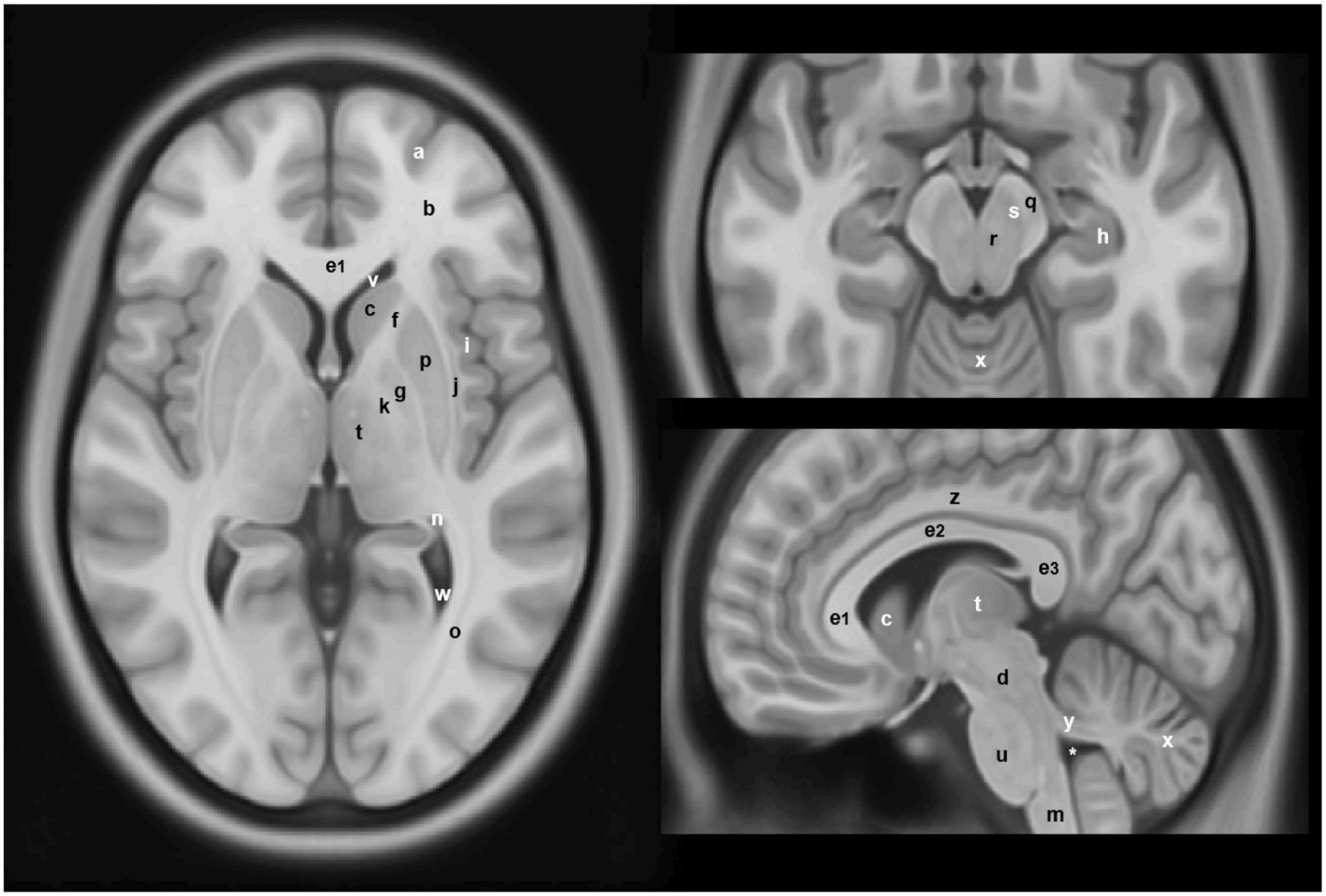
Anatomical locations of some of the structures and regions important in Parkinson's disease and atypical Parkinsonian syndromes, highlighted on a standard averaged T1-weighted MNI template for normal population. Labeling: a = cerebral gray matter (frontal lobe), b = cerebral white matter (frontal lobe), c = head of caudate nucleus, d = midbrain, e1 = genu of corpus callosum, e2 = body of corpus callosum, e3 = splenium of corpus callosum, f = anterior limb of internal capsule, g = globus pallidus, h = hippocampus, i = insular cortex, j = claustrum, k = posterior limb of internal capsule, m = medulla oblongata, n = tail of caudate nucleus, o = optic radiation, p = putamen, q = crus cerebri (anterior portion of cerebral peduncle), r = red nucleus, s = substantia nigra, t = thalamus, u = pons, v = anterior horn of lateral ventricle, w = posterior horn of lateral ventricle, x = cerebellum, y = superior cerebellar peduncle, z = cingulate gyrus, * = fourth ventricle. Note: p and g together constitute the lentiform nucleus; c and p together constitute the dorsal striatum. The template was obtained from McConnell Brain Imaging Center, Montreal Neurological Institute, McGill University Copyright 1993–2004 Fonov et al. (33).
